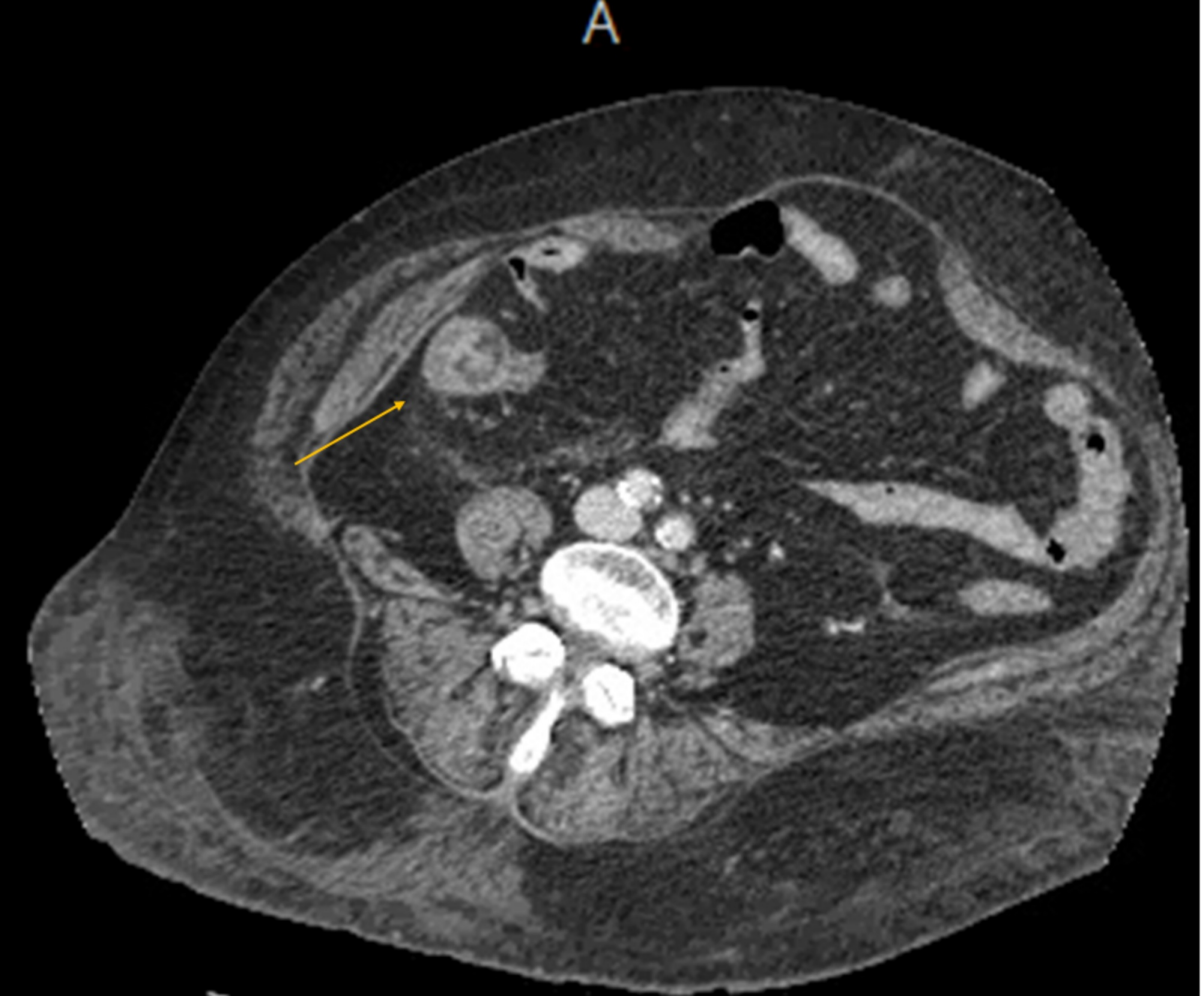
CT scan showing colitis, most prominent in the right colon (arrow).
